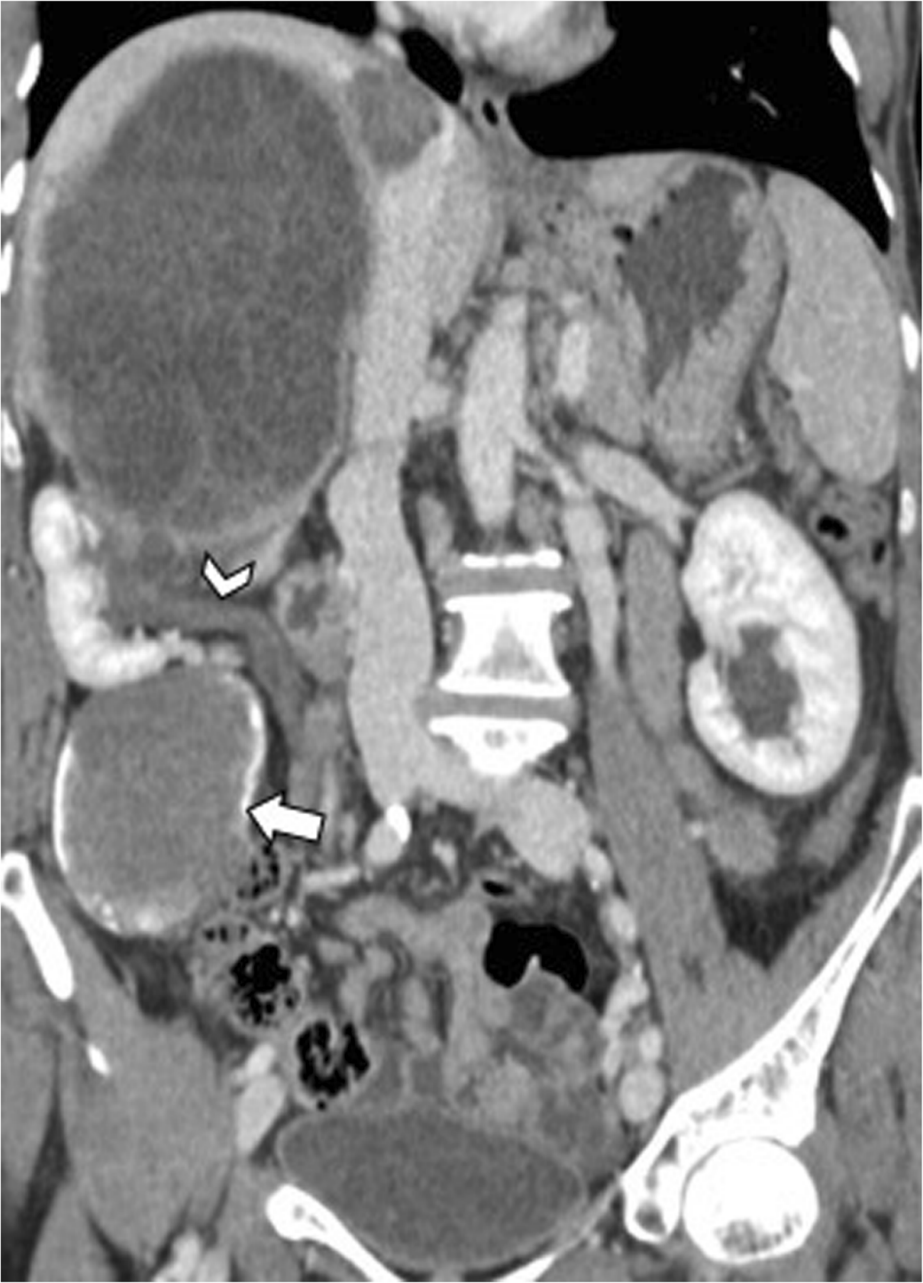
A 60-year-old woman with large partially exophytic hepatic hydatid cyst. Coronal reformatted CT image on portal venous phase shows a large hepatic hydatid cyst with multiple daughter cysts, associated with peritoneal hydatid cysts (arrow). The exophytic growth of the hepatic hydatid cyst causes significant mass effect on the right kidney, which appears compressed and dislocated with hydronephrosis (arrowhead)
